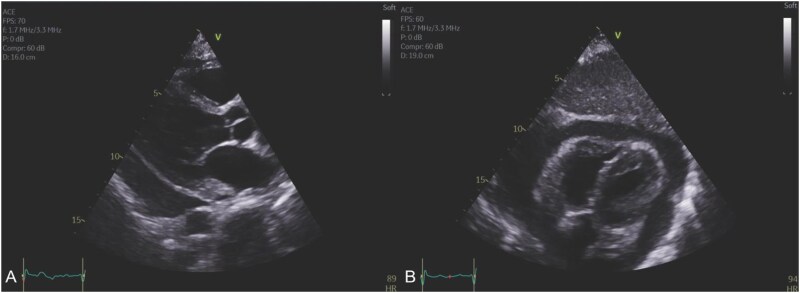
Transthoracic echocardiogram. (*A*) Parasternal long-axis image demonstrating a large pericardial effusion. (*B*) Subcostal image demonstrating early right ventricular diastolic collapse in the context of the large circumferential effusion. Supplementary material online, videos available.
